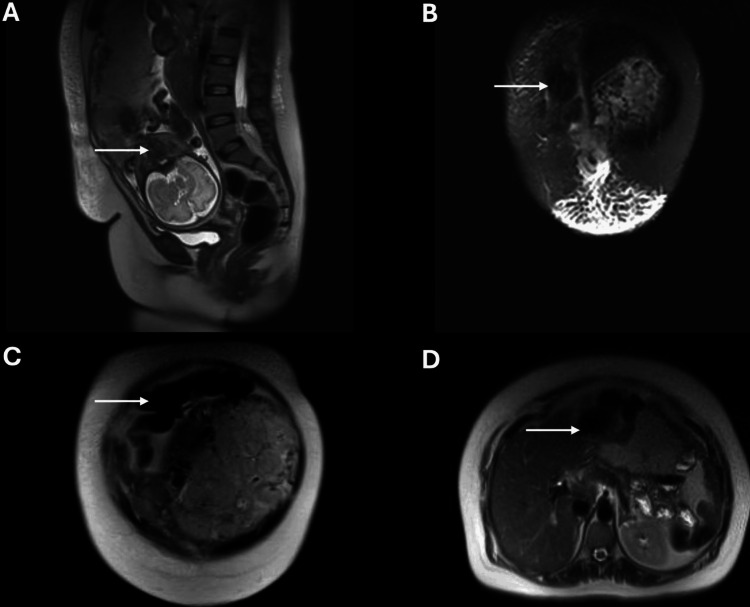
Magnetic resonance imaging (MRI) of the abdomen and pelvis showing a right-sided rectus sheath hematoma in a 33-week pregnant patient. (A) Sagittal T2-weighted image showing the hematoma compressing the anterior abdominal wall and the gravid uterus inferiorly. (B,C) Axial T2-weighted images showing a heterogeneous hyperintense lesion within the right rectus sheath. (D) Axial T1-weighted image demonstrating a hypointense region consistent with an organized hematoma, without signs of active bleeding.
